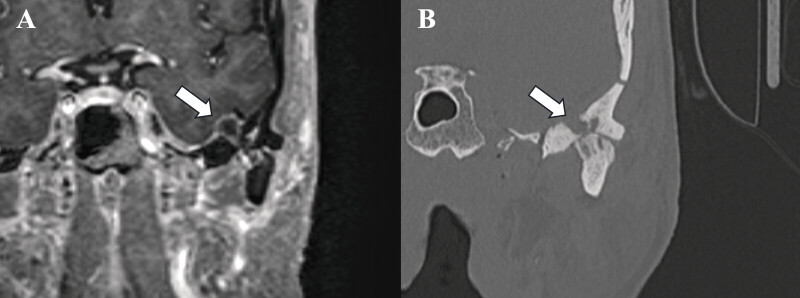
Case 2 coronal postcontrast T1-weighted MR image (
